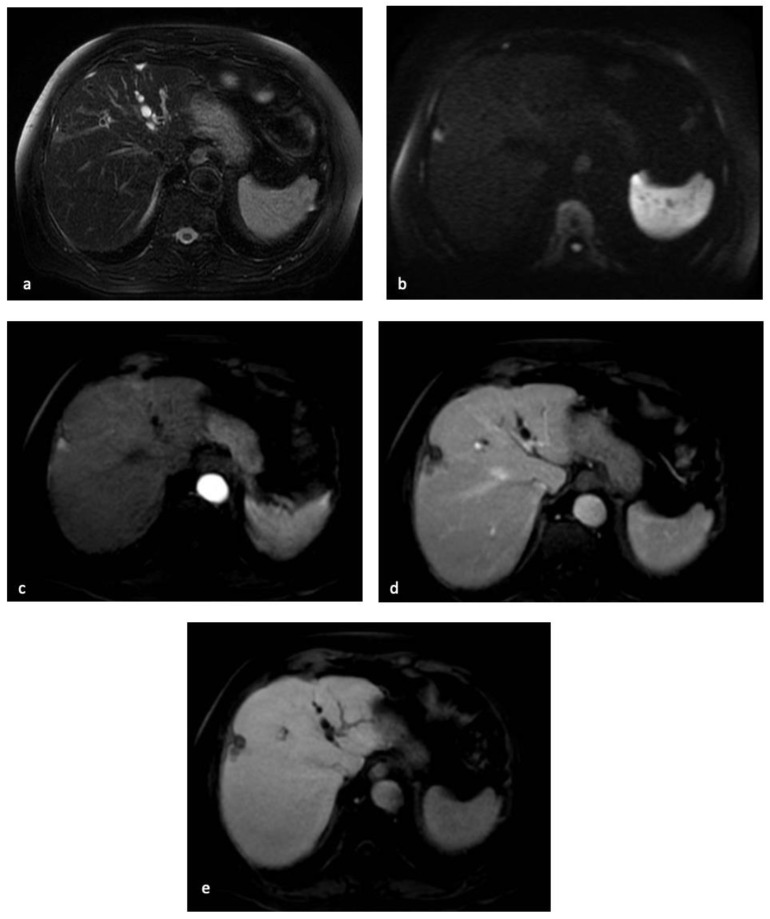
MR examination of the patient discussed in Figure 1 and Figure 2. On T2-weighted images, the centimetric subcapsular appeared as hyperintense (a). On DWI with a b-value of 1000, the lesion showed signal restriction (b). After administration of a hepatobiliary contrast agent, the lesion showed arterial hyperenhancement (c) with hypointensity in the portal-venous phase (d) and in the hepatobiliary phase. (e) The lesion appeared hypointense.
